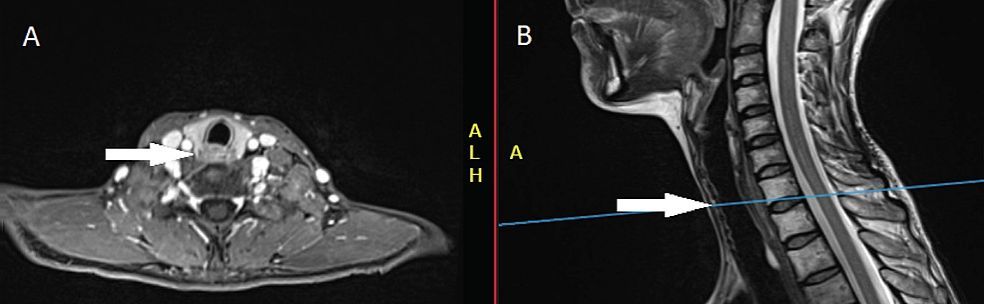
Post-treatment Imaging performed six weeks after completing the chemoradiation Axial (A) and sagittal (B) T1-weighted MRI shows a complete response to the treatment.
